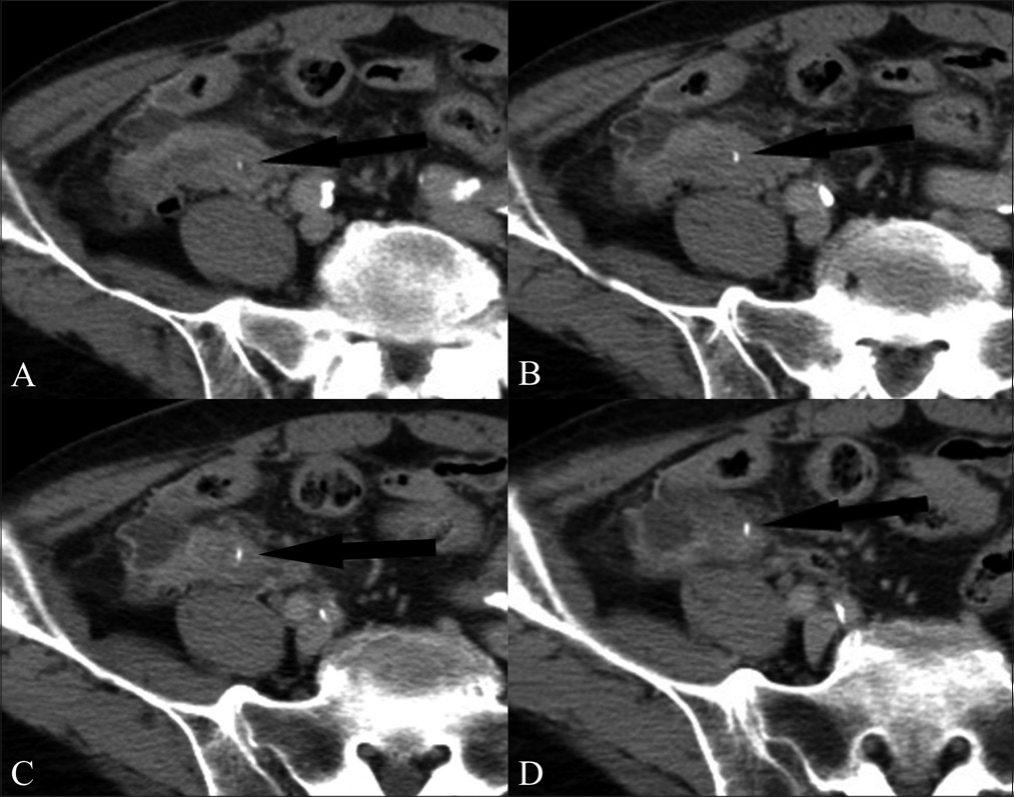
Sequential axial contrast-enhanced CT scans through the lower abdomen show a linear radiodense FB (arrow) in the distal ileum. It can be seen to traverse the thickened bowel wall. Surrounding stranding of the mesenteric fat is noted. There was no pneumoperitoneum
